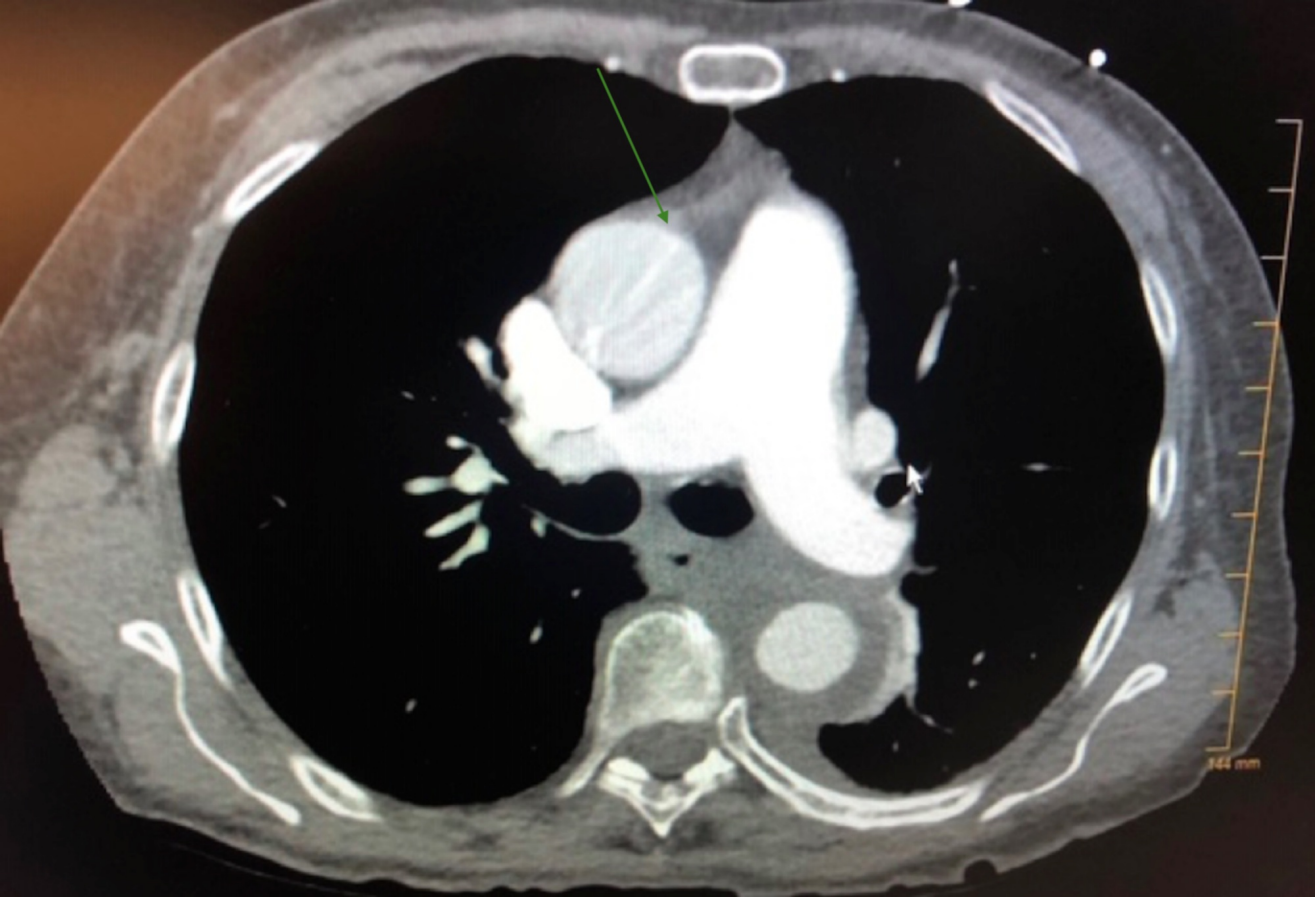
Aortic dissection with extravasation CT scan of the chest with intravenous contrast showing aortic dissection with extravasation.
